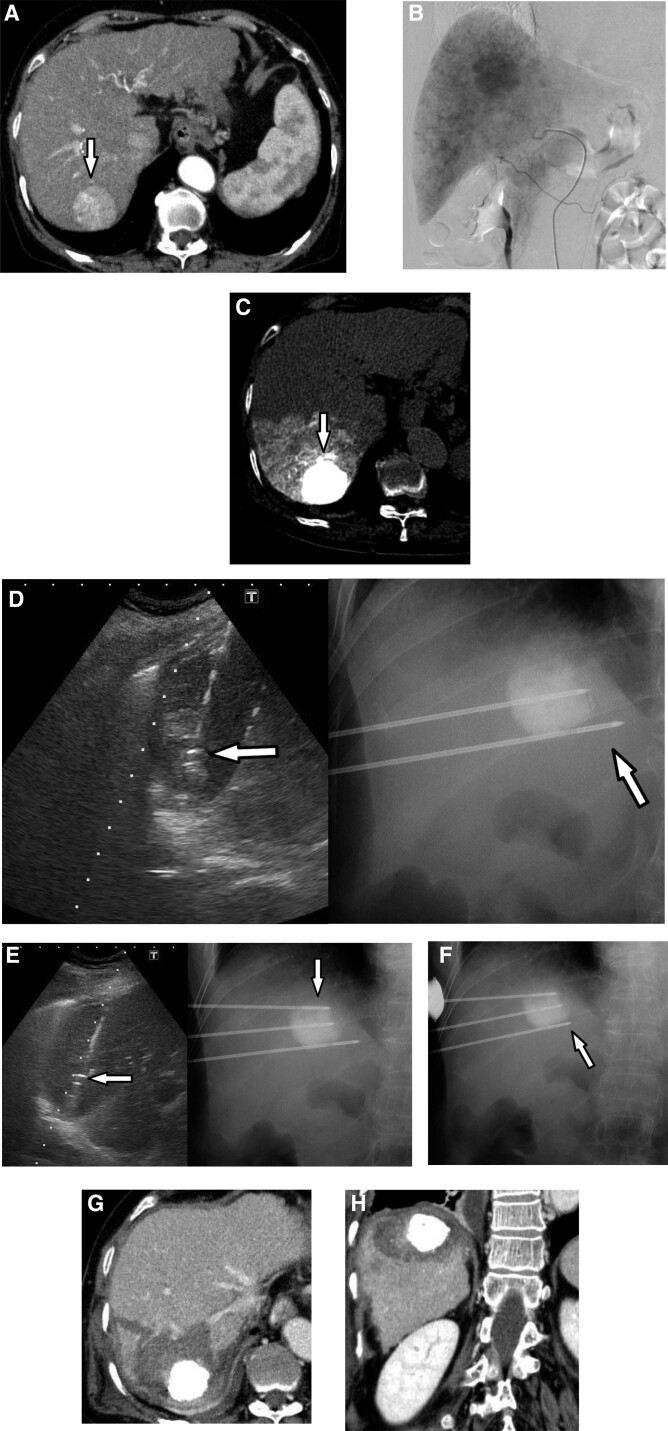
(A–H) Multipolar RFA treatment assisted with C-arm X-ray fluoroscopy imaging. A 74-year-old female patient with 4.3 cm right-lobar HCC (Segment 7). Twelve days post-TACE, multipolar RFA using 3 bipolar electrodes was performed. (A) The tumor shows hypervascularity in the early phase with dynamic CT imaging (white arrow). (B) Angiography and TACE was performed. (C) Remarkable epirubicin plus lipiodol emulsion was accumulated into the tumor (white arrow). (D, and E) Since the tumor outline is clear on fluoroscopy images, safe and appropriate insertion is achieved by synchronizing the US and fluoroscopic image (white arrows). (F) Immediately before ablation, the electrode is easily adjustable via reflecting fluoroscopic image (white arrow). Superiority over CT-guided RFA implies achieving proper insertion by real-time synchronization of the US and X-ray fluoroscopy images. (G and H) Portal venous phase axial and coronal CT images 3 days post-RFA show HCC with iodized oil retention and non-enhancing peri-tumor area, indicating technical success. CT = computed tomography, HCC = hepatocellular carcinoma, TACE = Transcatheter arterial chemoembolization, RFA = Radiofrequency ablation, US = ultrasonography.
